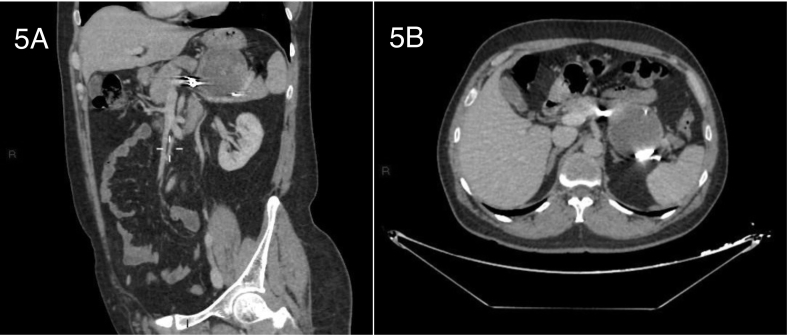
CT images in delayed phase. 5A: CT angiography in coronal cut, showing no flow in the pseudoaneurysm 7 months later. 5B: CT angiography in axial cut, showing no flow 7 months later.
